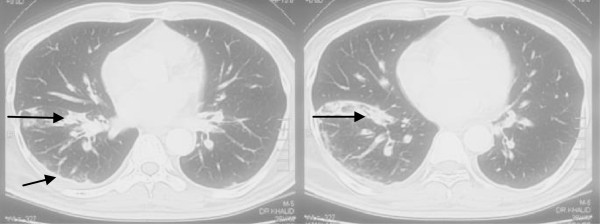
(Case 3) CT Chest before treatment for Nocardia (Consolidation right anterobasal segment & pleural effusion).
